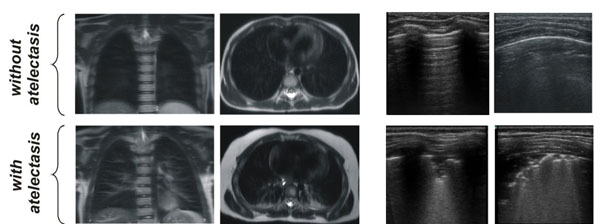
Example of patients with and without atelectasis. Left: MRI coronal and axial images used as reference method to detect anesthesia-induced atelectasis. Right: Atelectasis induced by anesthesia is determined by LUS as irregular pleural line and subpleural consolidation with air bronchograms and trailing B lines.
